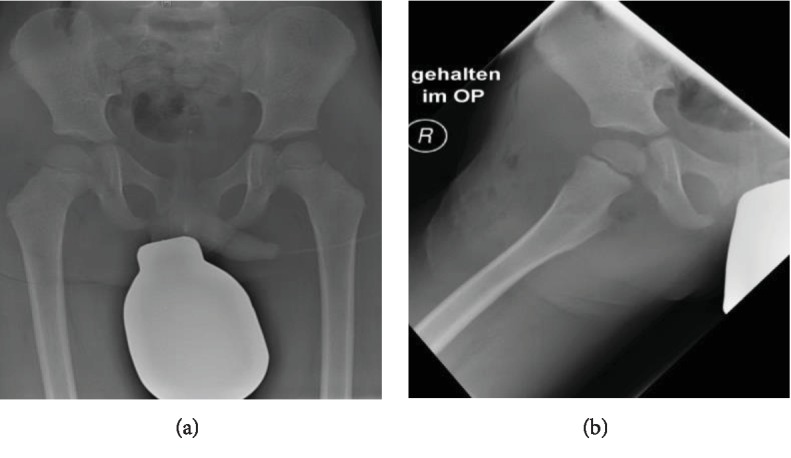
(a, b) Intraoperative X-rays showing the complete reduction in a.p. (a) and axial (b) view.
